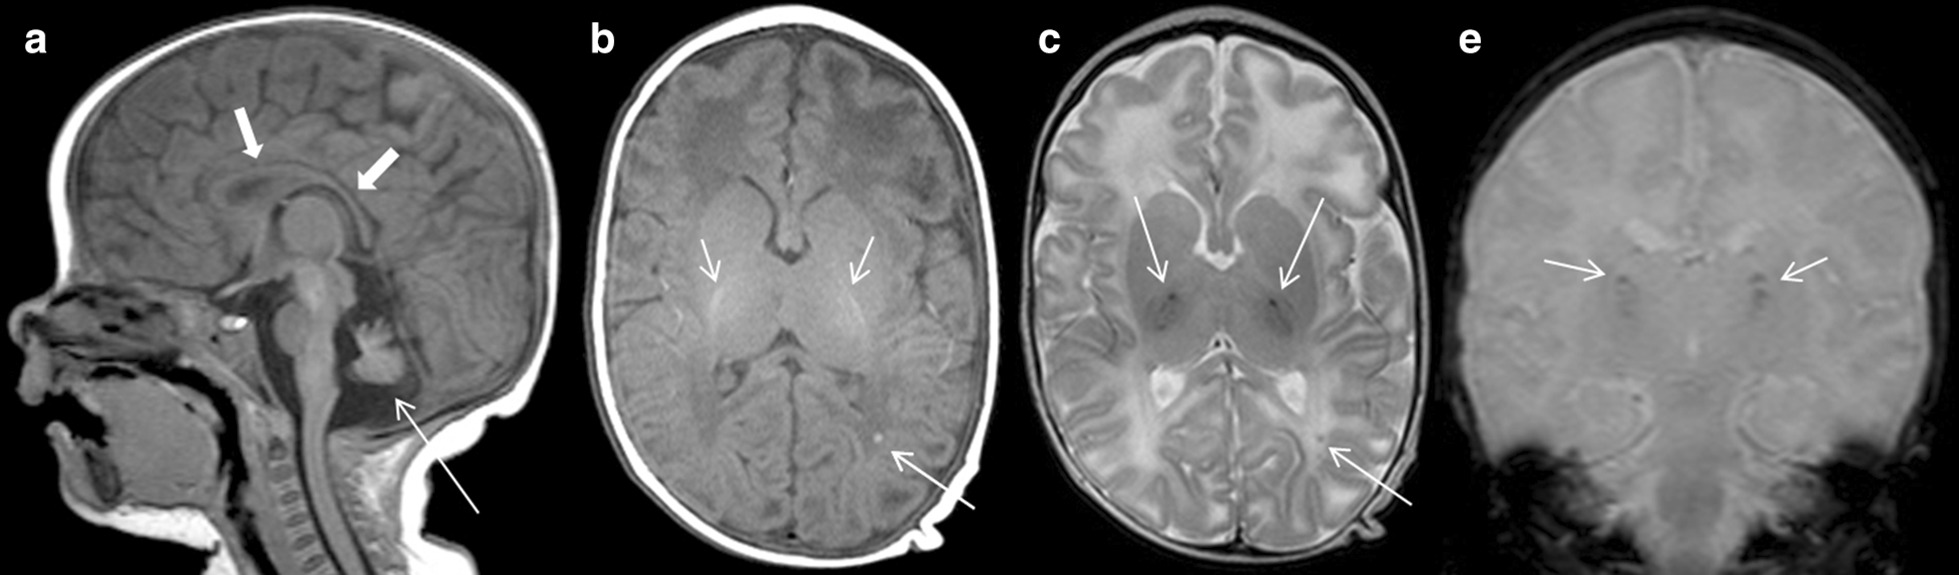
Initial MRI at the age of 2.5 months performed after the first partial seizure. Sagittal T1-weighted MRI image demonstrates cerebellar hypoplasia with an emphasis on the vermis and tonsils (arrow) and consecutive enlargement of the cerebrospinal fluid (CSF) spaces. The corpus callosum has a normal shape and size (thick arrow) (a). Transversal T1-weighted MRI image shows normal myelination within the internal capsule concealing the punctuate high signal (b) corresponding to the low signal areas in the T2-weighted (c) and T2*-weighted images within the thalami (d). These areas of signal abnormality correspond to calcifications. Also note the abnormal signal intensity in the frontal lobes (b, c) corresponding to leukencephalopathic areas also seen in the follow ups. At this point there was no administration of gadolinium contrast material
